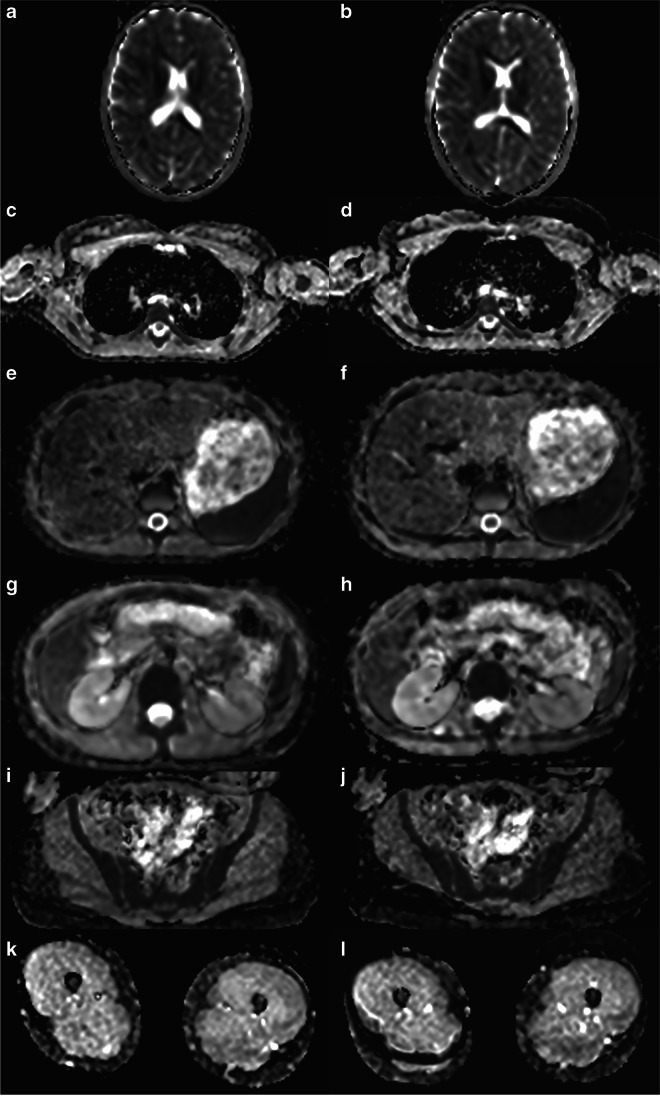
Comparison of axial orientated ADC (apparent diffusion coefficient) parameter map for brain (a,b), chest (c,d), upper abdomen (e,f), kidneys (g,h), pelvis (i,j) and thigh muscles (k,l) for the same 16-year-old girl shown in Fig. 1 with fever of unknown origin. a,c,e,g,i,k Standard diffusion-weighted imaging (DWI), b,d,f,h,j,l Simultaneous multi-slice-DWI. Overall comparable image quality
